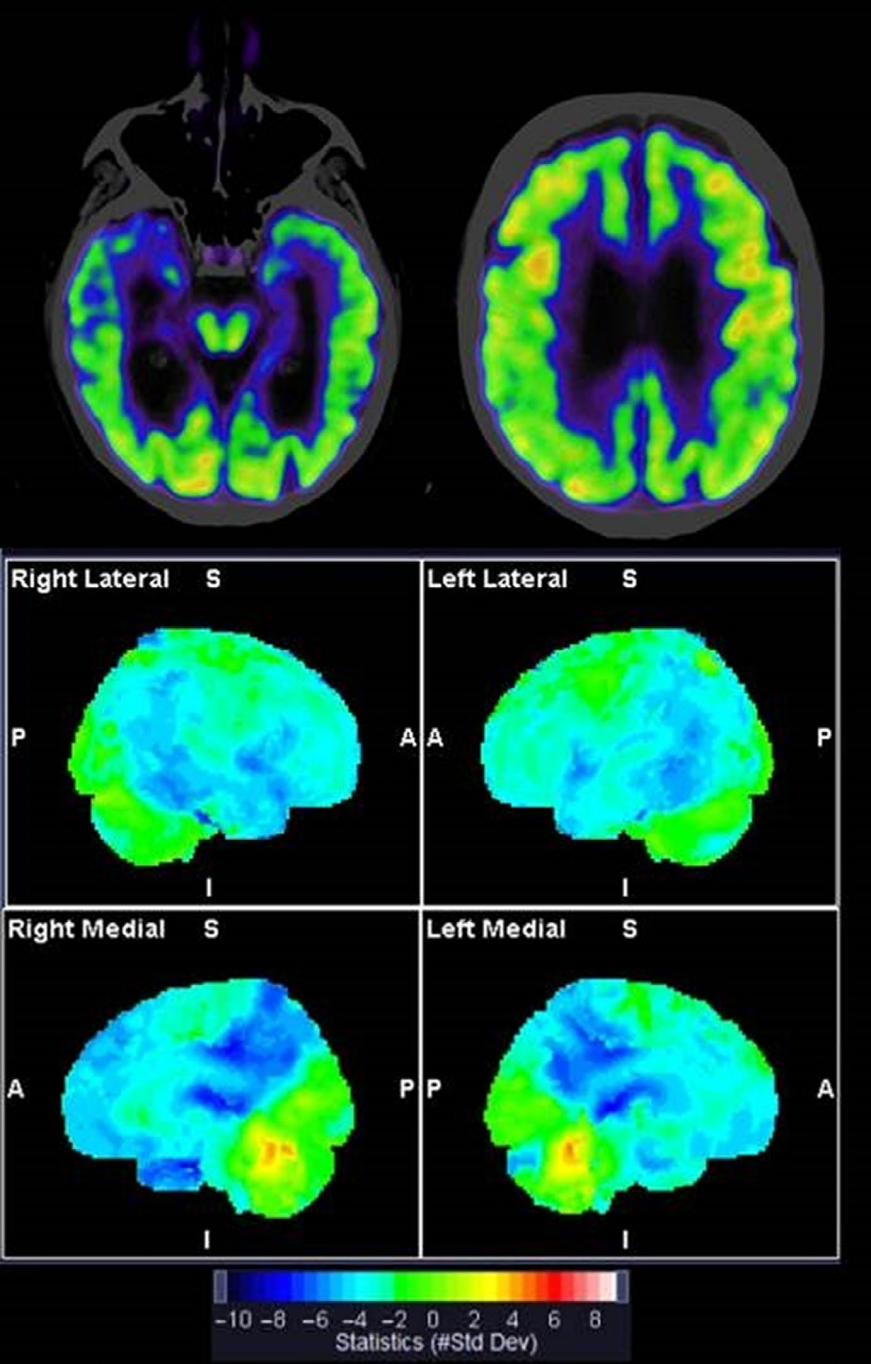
Brain [^18^F]FDG‐PET. Top row shows fused PET and CT axial sections at the level of mesial temporal lobe and posterior cingulate gyrus. Bilateral reductions are present. Below: statistical surface projections normalized to cerebellum and vermis (Syngo.via software, Siemens‐Healthineers). The color bar indicates the standard deviations relative to healthy subjects age 46‐79 y. Characteristic AD‐like pattern with hypometabolism in parietotemporal cortex, posterior cingulate gyrus, and precuneus is demonstrated
